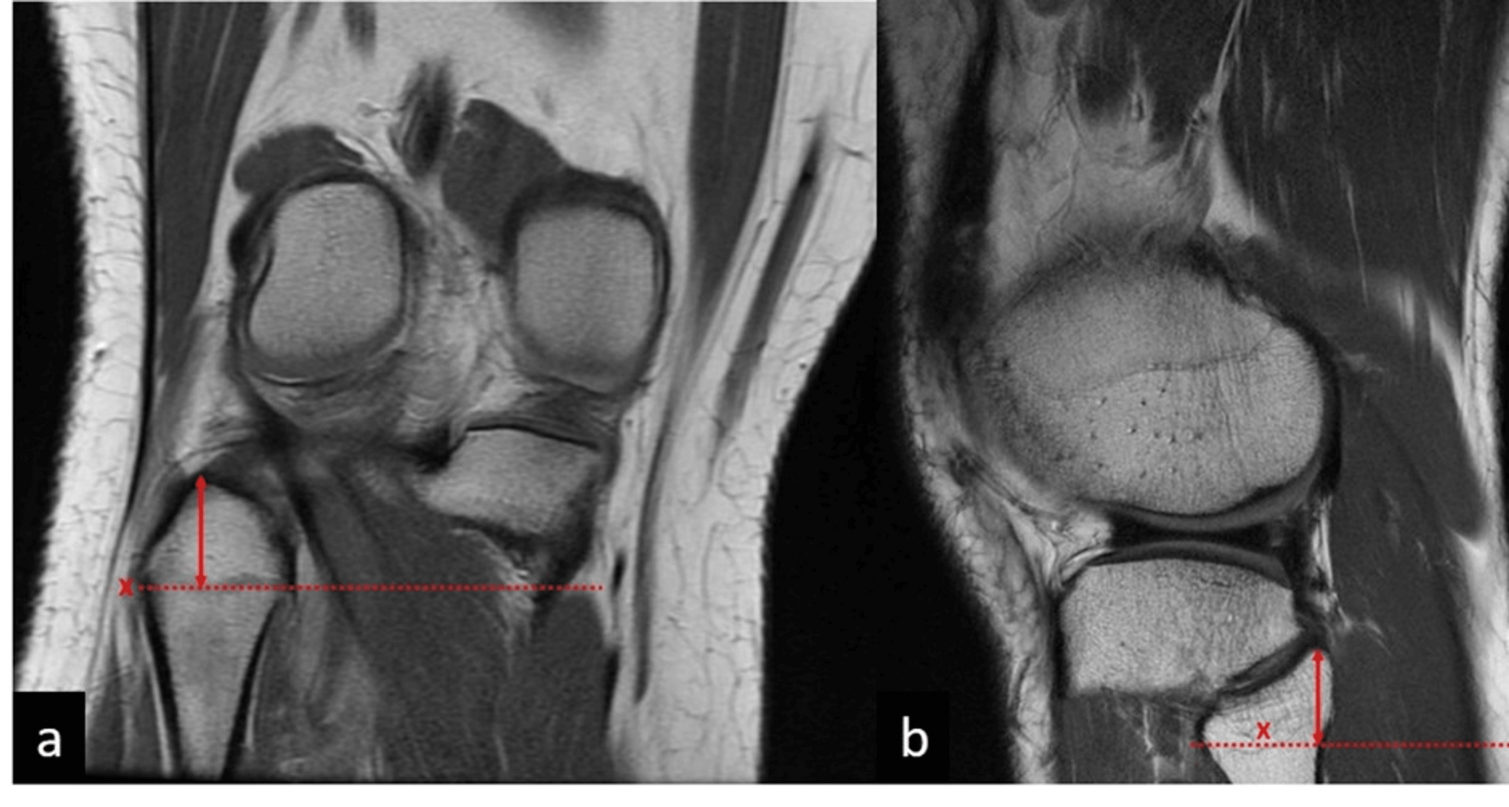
MRI imaging of the right knee in the coronal (a) and saggital (b) views. The distance between the tip of the fibula head and the pre-determined entry point (marked x), demarcated in arrowed red lines is measured.
